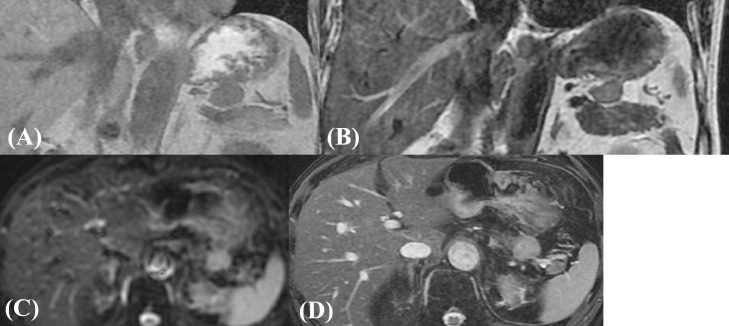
MRI findings (A) T1WI, (B) T2WI, (C) DWI, (D) FIESTA MRI revealed a well-defined mass that had iso-intensity to muscle on T1WI, slight hyper-intensity to muscle on T2WI, and slight hyperintensity on DWI. There was adipose intervening between the tumor and the stomach or pancreas.
